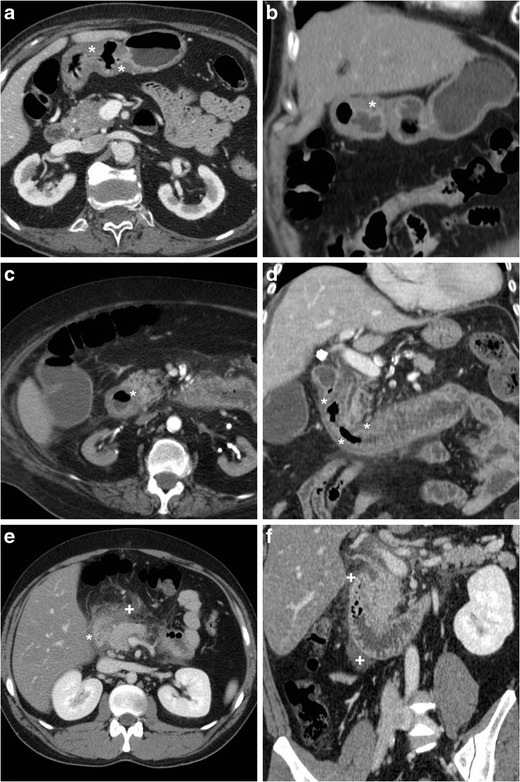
In a 34-year-old female with unspecific abdominal pain and unremarkable endoscopic findings, the contracted gastric antrum showed at CT (a, b) apparent hypoattenuating mural thickening (*), which mimicked characteristic appearance of acute peptic disease but interrupted at the site of spasms and lacked mucosal hyperenhancement. Non-inflammatory circumferential oedematous mural thickening (*) of the proximal and descending duodenum is seen in a 43-year-old female with anasarca due to renal failure (c, d). In a 29-year-old alcohol-addicted male with interstitial oedematous acute pancreatitis, contrast-enhanced CT (e, f) showed normally enhancing pancreas, ill-defined oedematous duodenal wall (*) with associated periduodenal fluid and fat stranding (+) extending from the proximal to the third duodenum
